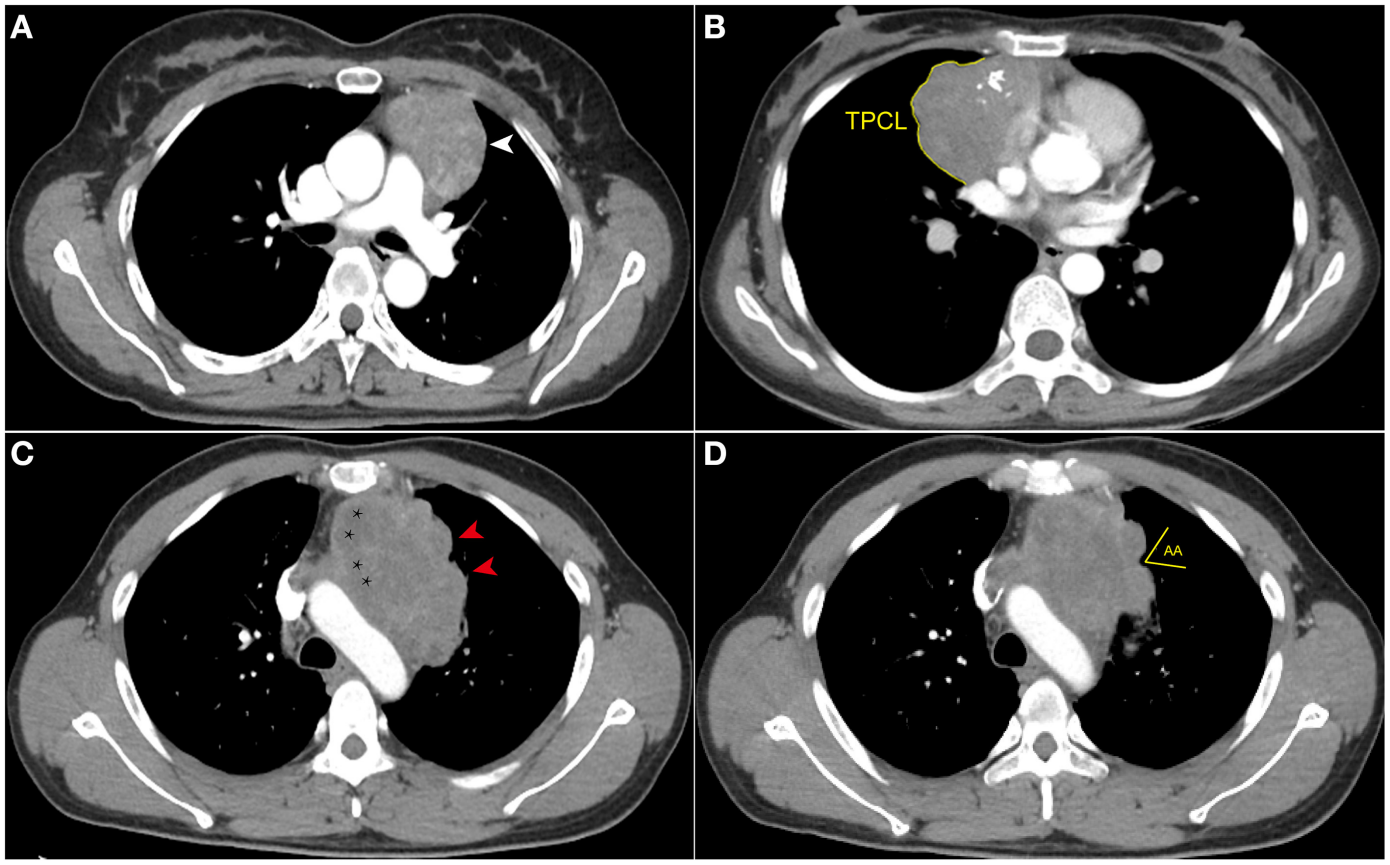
Computed tomography imaging evaluation of contour, internal density, and measurement of the new index. (A) Axial contrast-enhanced chest CT image obtained at the level of the pulmonary trunk demonstrated a thymoma with smooth contour (white arrowhead) in a 42-year-old woman (WHO classification AB). (B) Axial contrast-enhanced chest CT image showed a thymoma (WHO classification B1) in a 54-year-old woman. The multilobulated contour and internal calcifications were seen, and the tumor perimeter that contacted the lung (TPCL) was measured along the margin between tumor and adjacent lung (yellow arc line). (C) Axial contrast-enhanced chest CT image obtained at the level of the aortic arch demonstrated a thymoma (WHO classification B2) in a 49-year-old man who complained of chest distension and chest pain. The tumor has several obtuse angles formed by adjacent lobulations (red arrowhead), and low-density cystic or necrotic areas are indicated by stars. (D) In the same patient as (C), the acute angle (AA) formed by adjacent lobulations can be seen in the upper slices.
